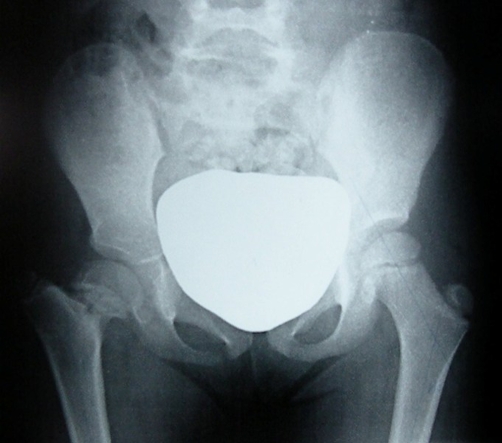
Anteroposterior radiograph of the pelvis shows coxa vara and growth arrest with the femoral neck shortening and an elevated greater trochanter.
